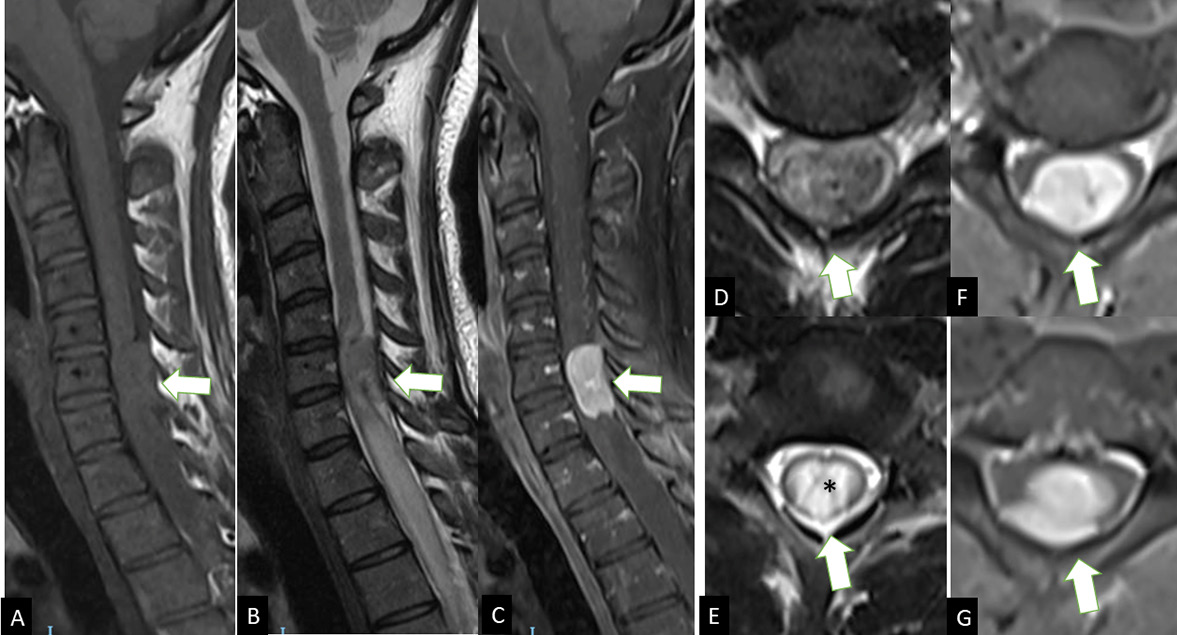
Conventional sagittal MRI images show an intradural and extramedullary
